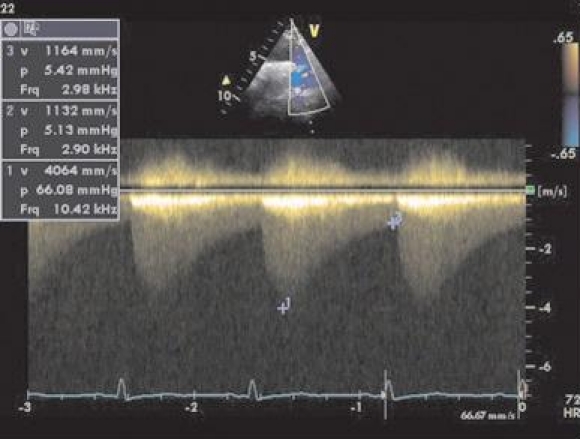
Aortic coarctation. 2D transthoracic echocardiography suprasternal section at patient with sever coarctation of aorta; the Doppler exam reveals a continuous flow with maximum of the velocity of 4,6 m/s.
